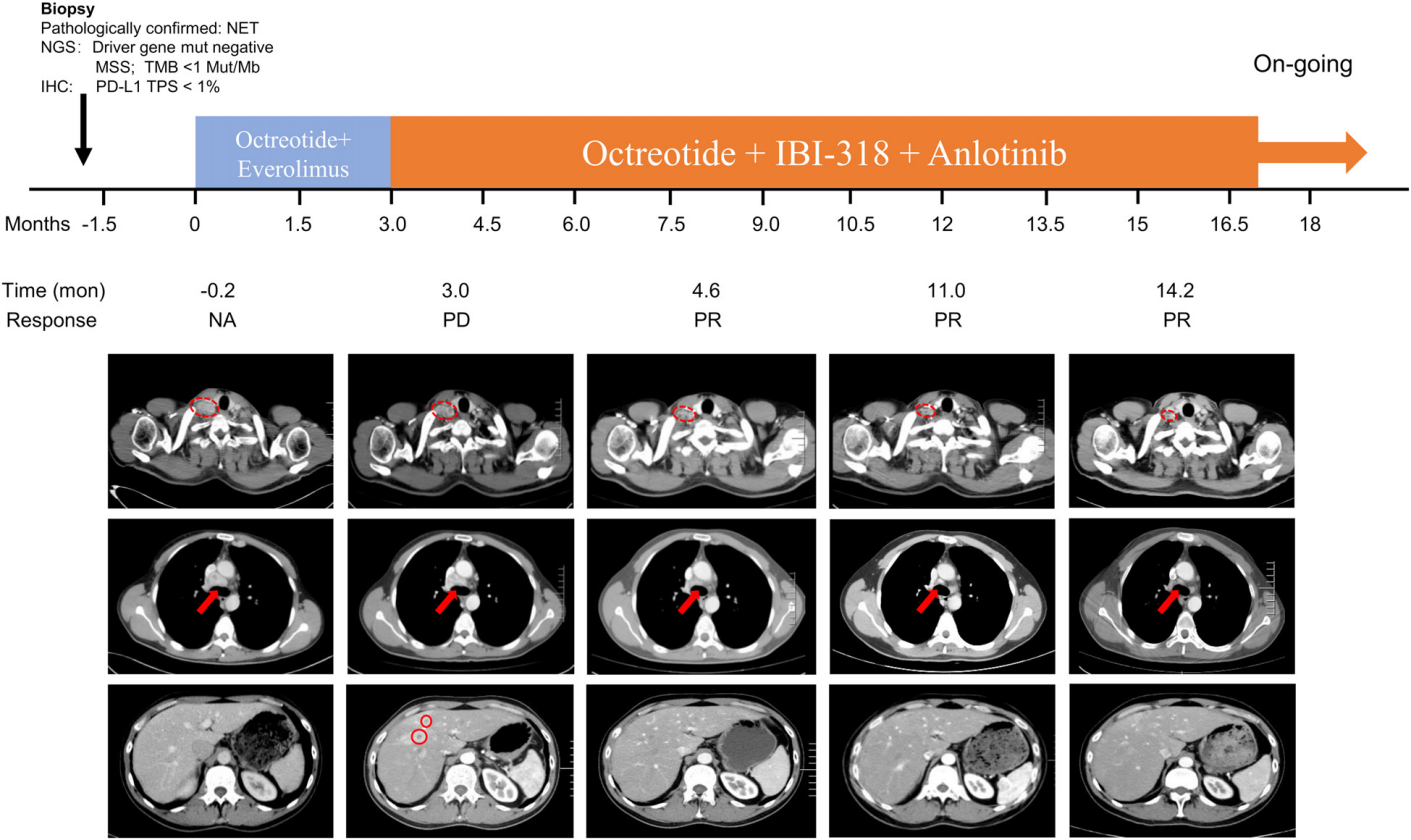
Timeline of the medication and computed tomography (CT) results before and during treatment. A pre-treatment enhanced CT scan was performed on April 11, 2022, showing multiple swollen lymph nodes in the right lower neck, bilateral supraclavicular areas, and left internal mammary area and mediastinum, and multiple low-density nodules in the liver. The first enhanced CT after starting treatment was on July 12, 2022, and showed that the multiple swollen lymph nodes in the right lower neck, bilateral supraclavicular areas, left internal mammary area, and mediastinum were smaller than before treatment, and multiple new ring-enhanced nodes appeared in the liver. The second enhanced CT scan after starting treatment was on August 30, 2022, and showed that the enlarged lymph nodes and liver nodules were smaller. The third enhanced CT scan after starting treatment was performed on March 15, 2023, and showed that the lymph nodes and liver lesions were stable. The fourth enhanced CT scan on June 20, 2023, showed that the lymph nodes were slightly smaller, while the liver lesions were roughly stable.
